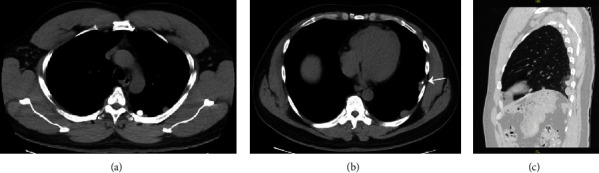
Computed tomography of the chest without contrast. (a) Axial view, mediastinal window at the level of the aortic arch reveals three pleural based nodules one of which is densely calcified, round, and smoothly marginated. (b) Axial view, mediastinal window of the lower thorax shows two pleural based smoothly marginated nodules of soft tissue density, one of which is partially calcified and lobulated (white arrow). (c) Sagittal view, lung window of left hemithorax showing four non-calcified pleural based nodules, one of which is lies along the left major fissure. Note the absence of the spleen.
